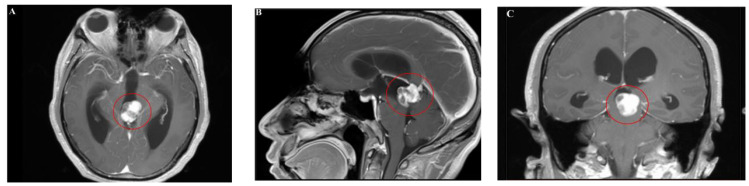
(A–C) Enhanced MRI showed marked heterogeneous enhancement.
